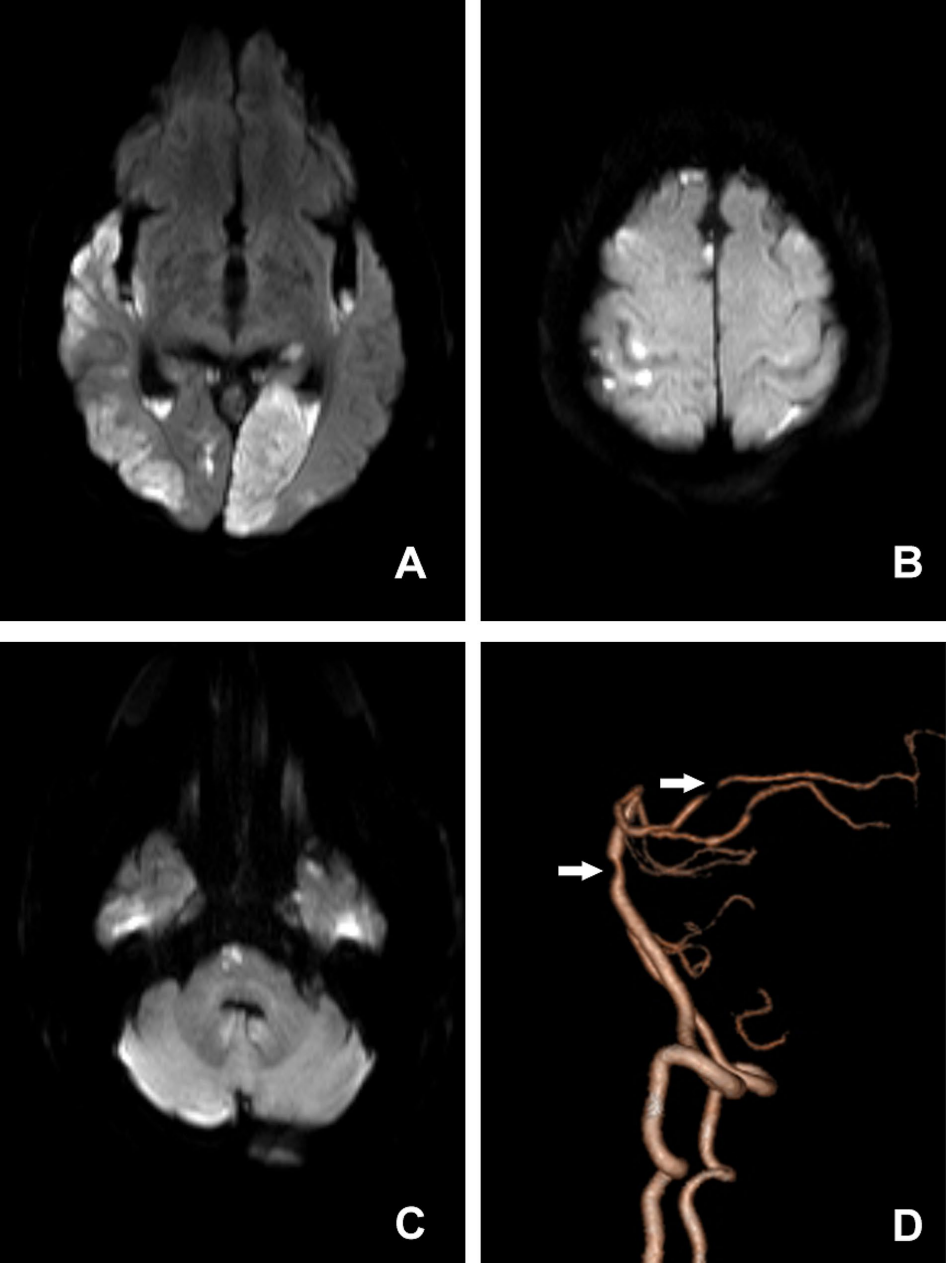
Imaging examination of the patient. Diffusion-weighted magnetic resonance imaging of the brain revealed high signals in the pons and bilateral frontotemporal parietal occipital lobes (A, B, C). Computed tomography angiography showed severe stenosis in the P2 segment of the posterior cerebral artery and moderate stenosis in the basilar artery (D)
